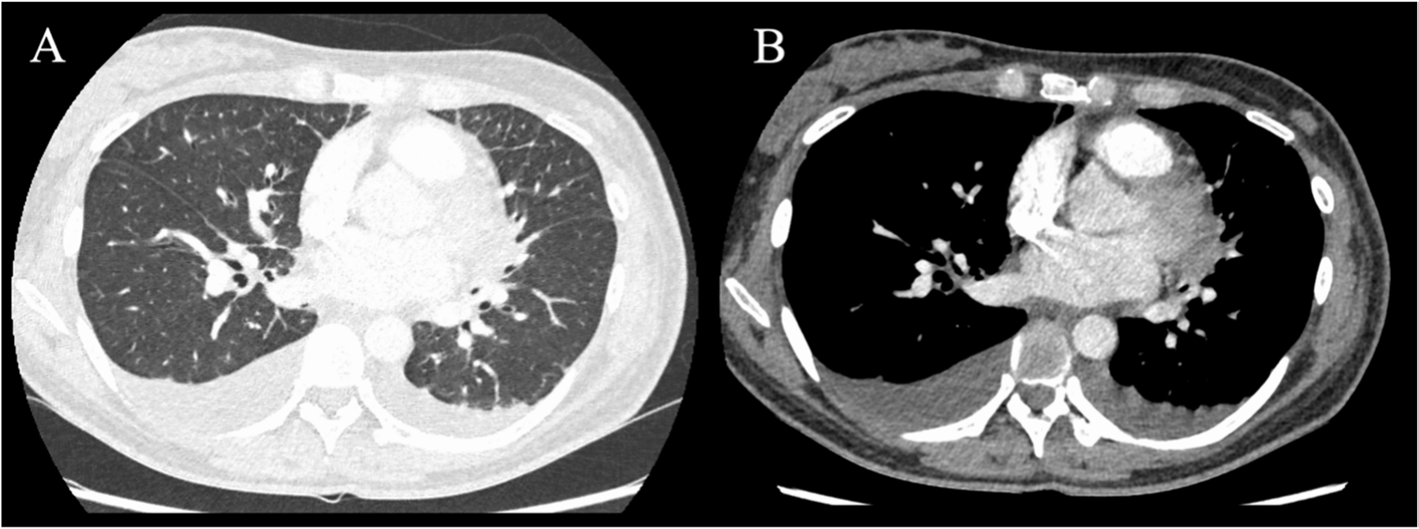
Computed Tomography on arrival at the hospital. A The chest CT (non-contrast) revealed bilateral pleural effusions, interlobular septal thickening in both lungs, and ground glass opacification in both lungs. The findings are consistent with pulmonary edema. B Contrast-enhanced CT showed no contrast defect in the pulmonary artery and only an enlarged uterus due to postpartum. There were no abnormal findings in other organs. CT, Computed Tomography
